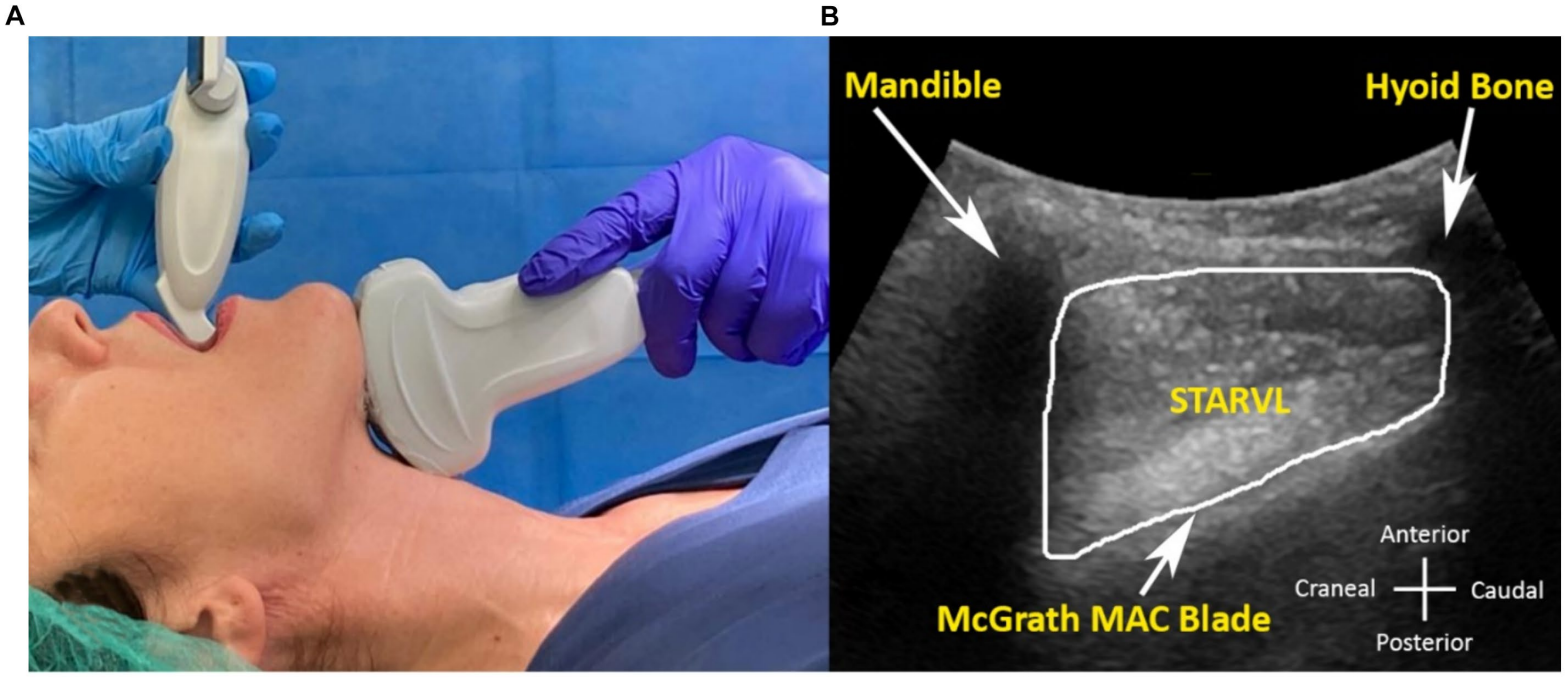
(A,B) Ultrasound image measurements in the anesthetized patient during videolaryngoscopy with McGrath^™^ Mac blade and the corresponding probe position. (B) Sagittal tongue area compressed by the videolaryngoscope (STARVL).
